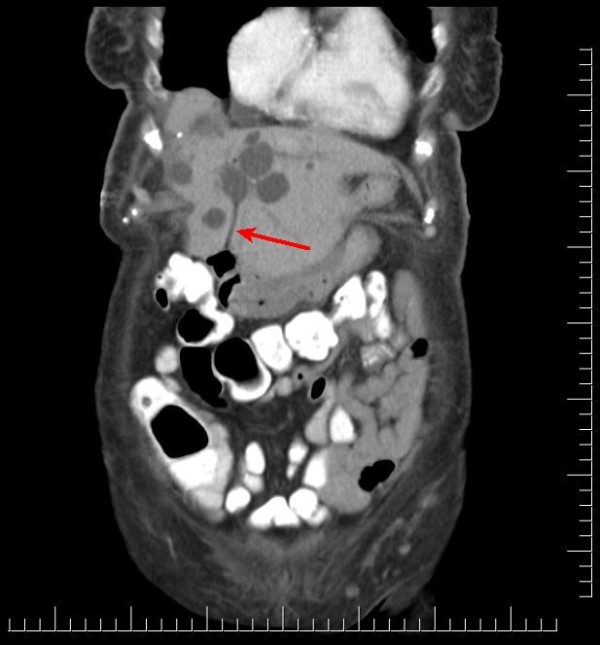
Coronal computed tomography showing hepatic cyst communication with the common bile duct.
